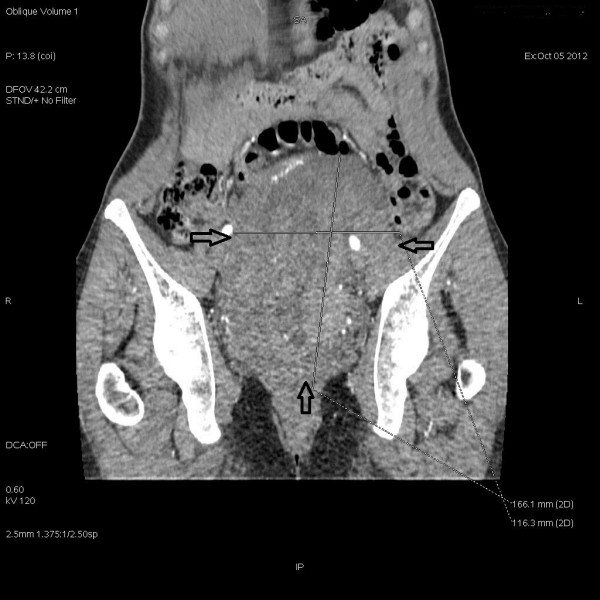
Coronal computed tomography scan of the pelvis. A large pathological tumour (arrows) filling up most of the minor pelvis.
